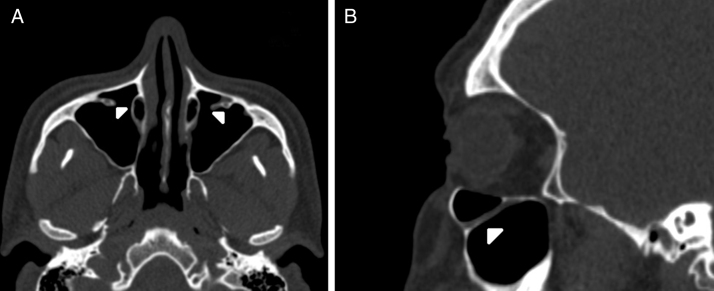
(A) Axial paranasal sinus CT image showing bilateral infraorbital canal Type 3 totally protruding into the maxillary sinus with a stalk (arrowheads). (B) Right parasagittal image showing infraorbital canal Type 3 (arrowhead).IOC Type 4, located anatomically at the outer limit of the zygomatic recess of the maxillary bone (lateroantral) (Fig. 4A and B).
